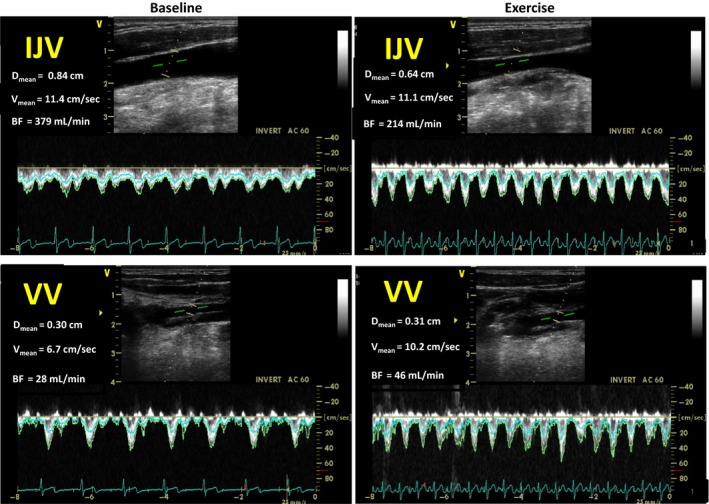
Doppler ultrasound images showing the internal jugular vein (IJV, upper panels) and vertebral vein (VV, lower panels) with Doppler flow waveform during baseline (left panels) and moderate exercise (right panels).
